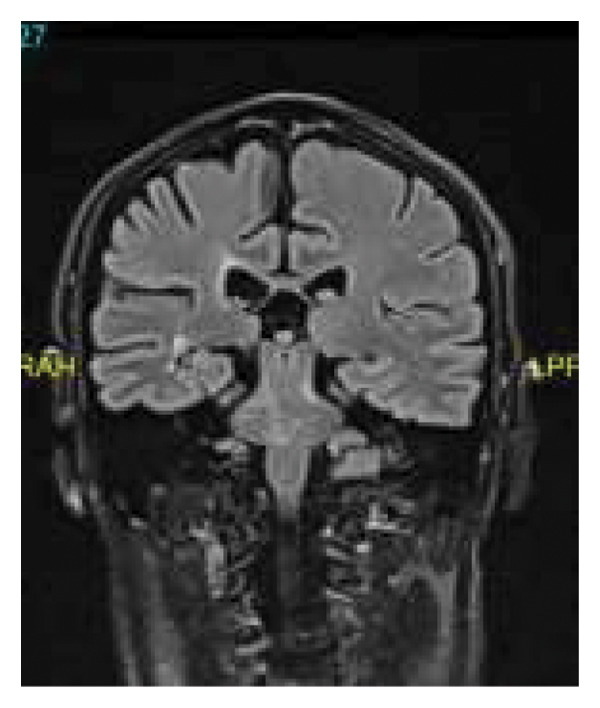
Coronal brain MRI, FLAIR sequence. Posttreatment radiological improvement.
